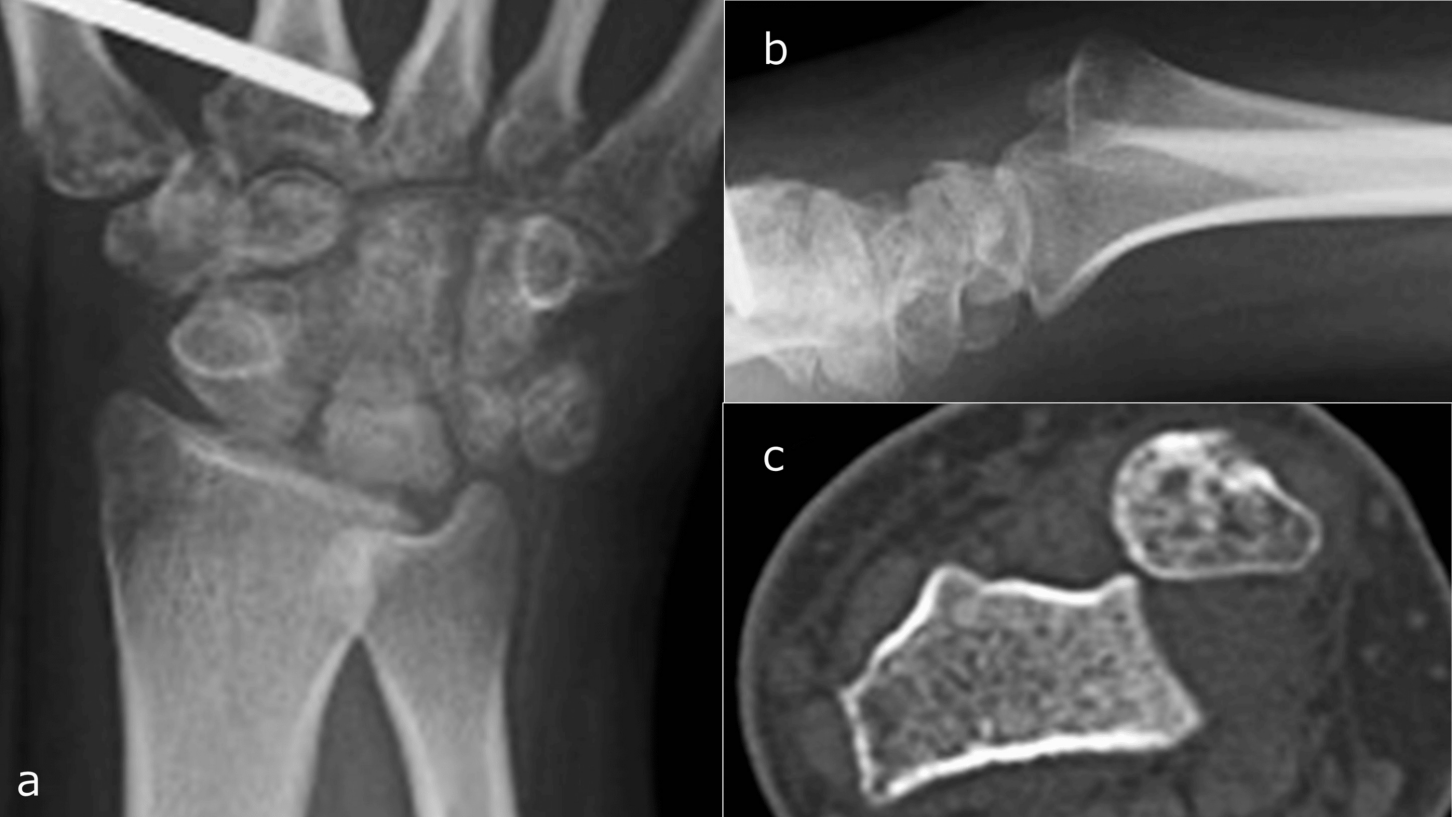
Post-second surgery imaging. a: X-ray anteroposterior view. b: X-ray lateral view. c: Computed tomography axial view.
